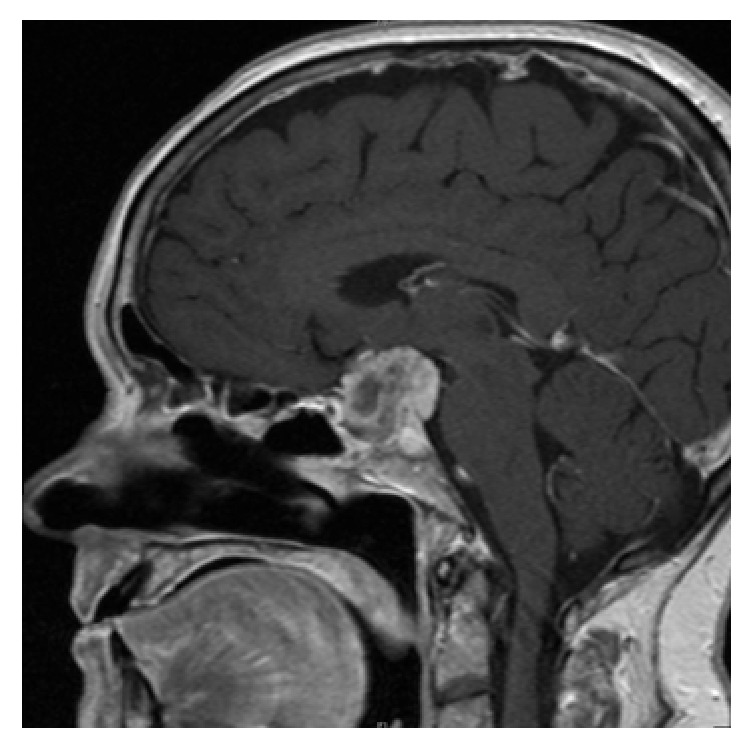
Postoperative sagittal MRI with contrast five weeks after surgery showing significant tumor recurrence.
